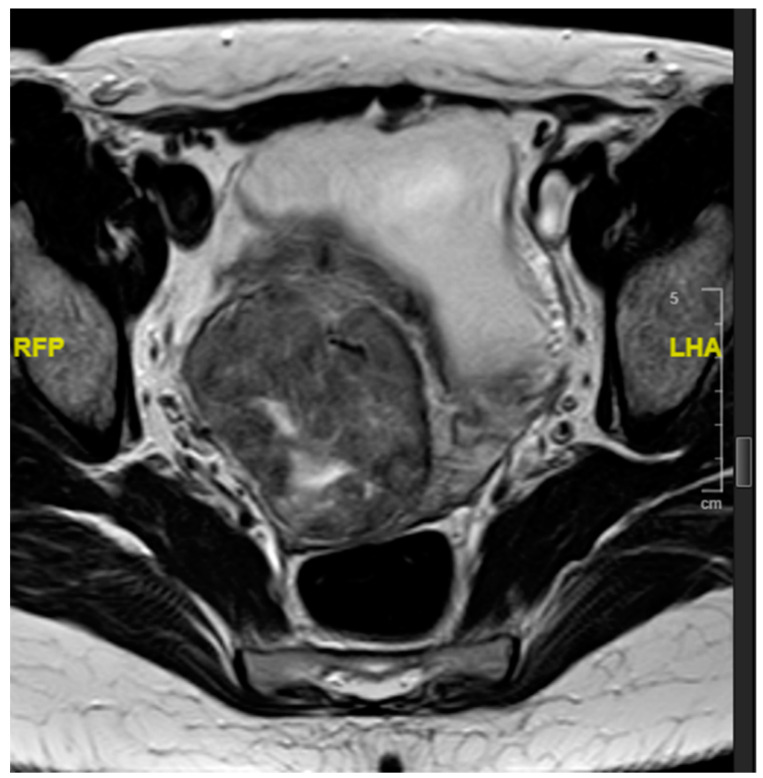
Transverse T2-weighted MRI surrounding the lower uterine cavity and distorting the uterine canal.
